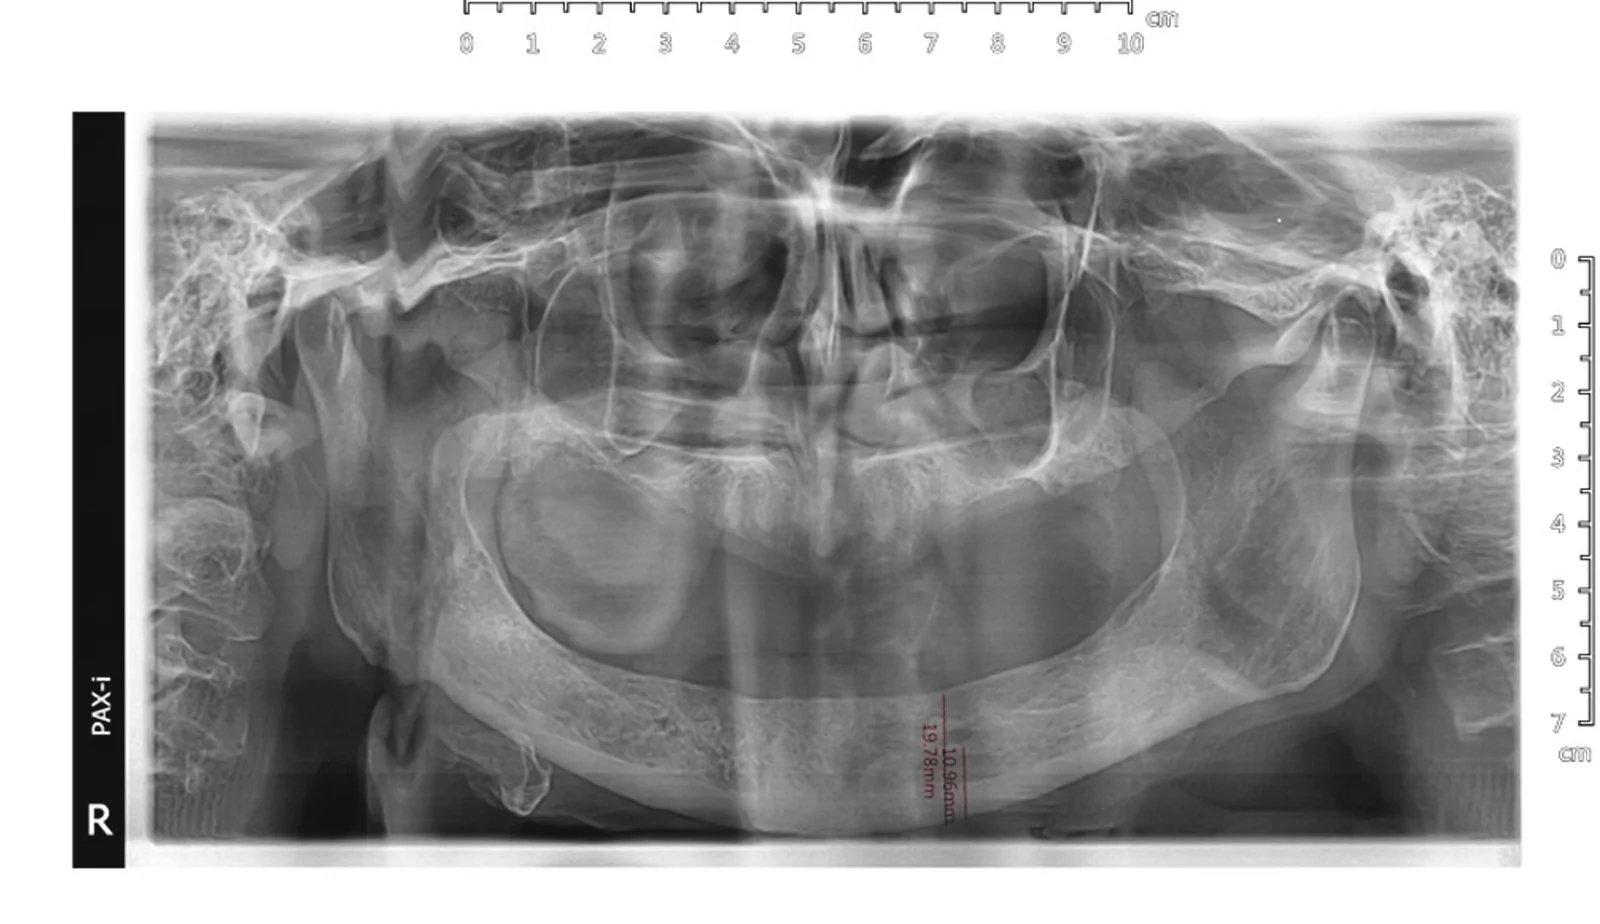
Orthopantomogram (OPG) showing linear measurement of mandibular residual ridge resorption
